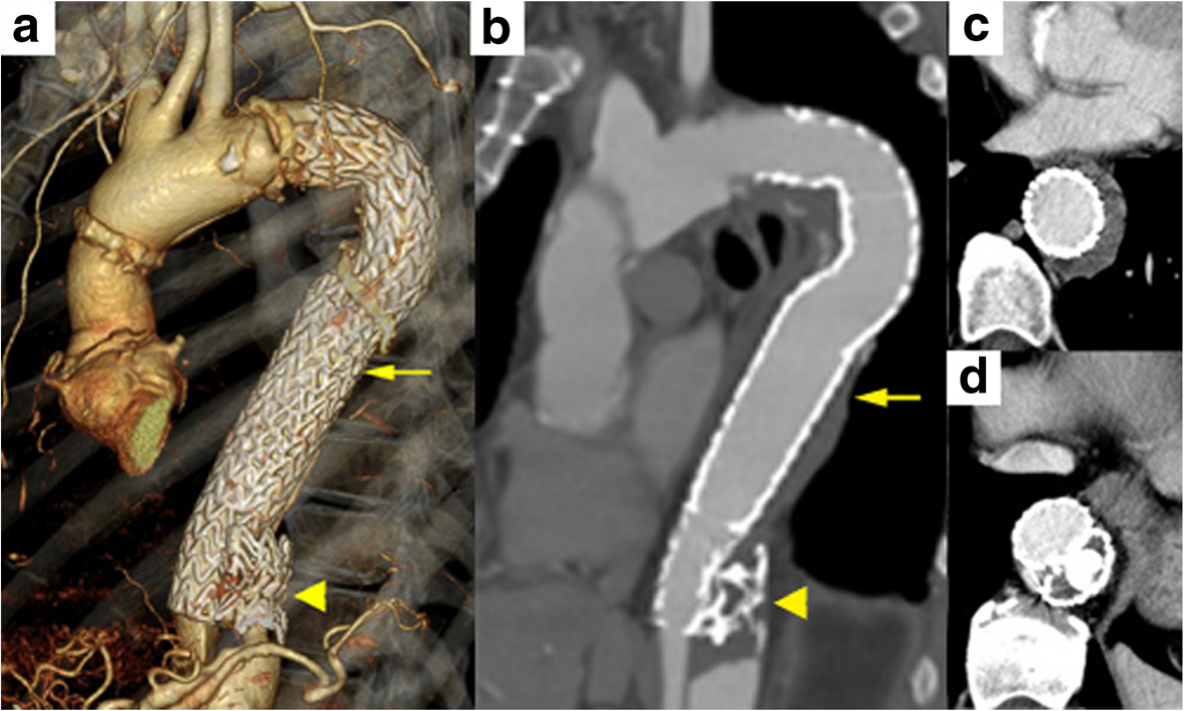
a Postoperative 3D–CTA imaging 1 year after TEVAR with implantation of a ‘candy-plug’. b A saggital slice showing the ‘candy-plug’ device with the Amplatzer vascular plug. c Decreased aneurysm diameter and false lumen thrombus formation (at the level of the arrow). d The ‘candy-plug’ with the Amplatzer vascular plug filling the central lumen (at the level of arrowhead)
